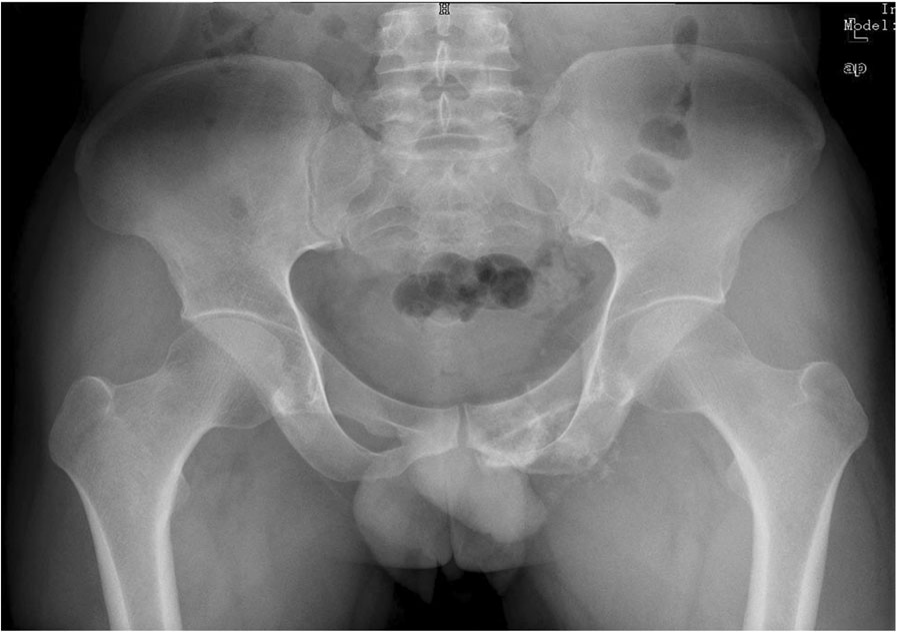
Pelvic X-ray in a patient with an chondrosarcoma (after biopsy) involving the superior and inferior pubis
